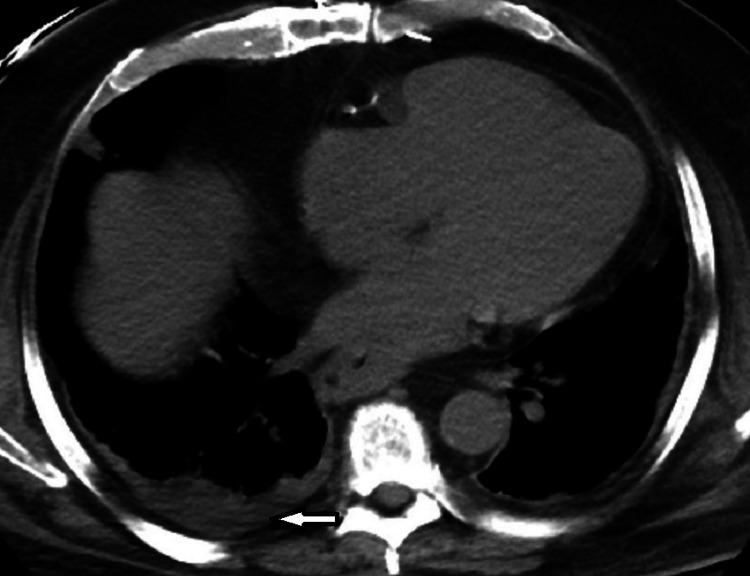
CT chest showing small right pleural effusion. CT: computerized tomography
